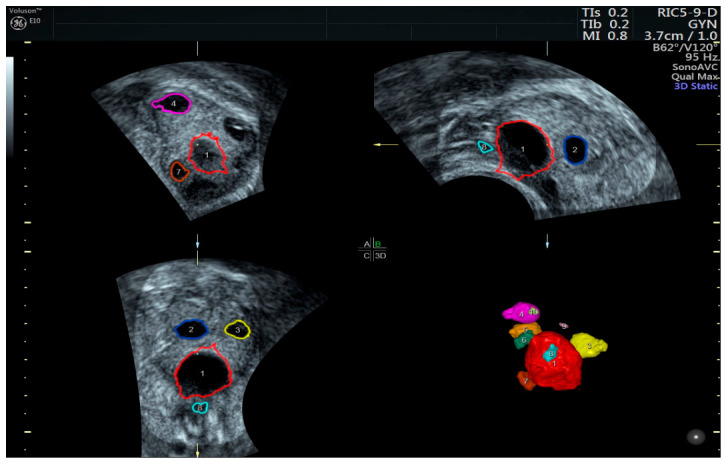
Representative three-dimensional ovarian ultrasound image obtained using the integrated SonoAVC™ (Sonography-based Automated Volume Count) software. (A) Plane A of the multiplanar display showing automatically detected antral follicles. (B) Plane B of the multiplanar display. (C) Plane C of the multiplanar display. (3D) Three-dimensional rendered reconstruction of the segmented follicles generated by SonoAVC. Different colors identify individual follicles automatically recognized by the software.
